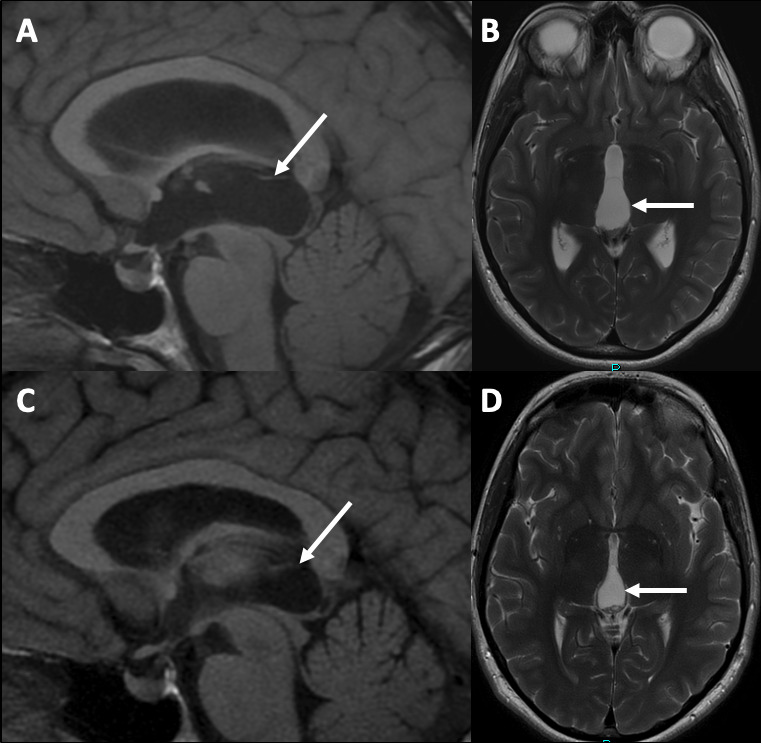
(A) Sagittal T1-weighted and (B) axial T2-weighted MRI sequences showing a large simple pineal region cyst (white arrows) causing symptomatic hydrocephalus at presentation. (C) Sagittal T1-weighted and (D) axial T2-weighted MRI sequences following endoscopic third ventriculostomy and cyst fenestration with collapse of the pineal region cyst and resolution of the hydrocephalus.
